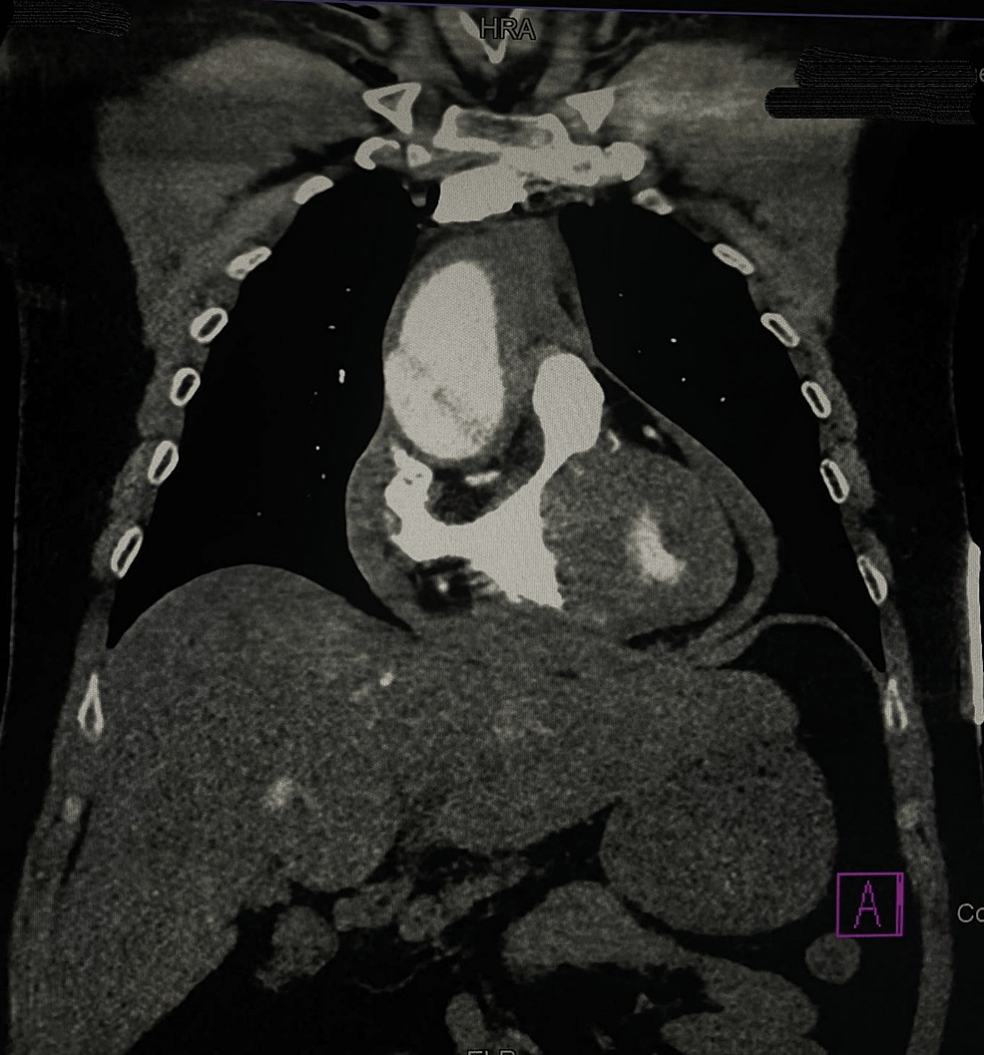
CT Angiography of Chest Computed tomography (CT) angiography of the chest showed ascending aortic ectasia, Type A, with focal rupture of the false lumen resulting in moderate pericardial effusion and mediastinum hematoma, the dissection flap raised 2 cm from the aortic valve. The coronary arteries arise from the true lumen.
